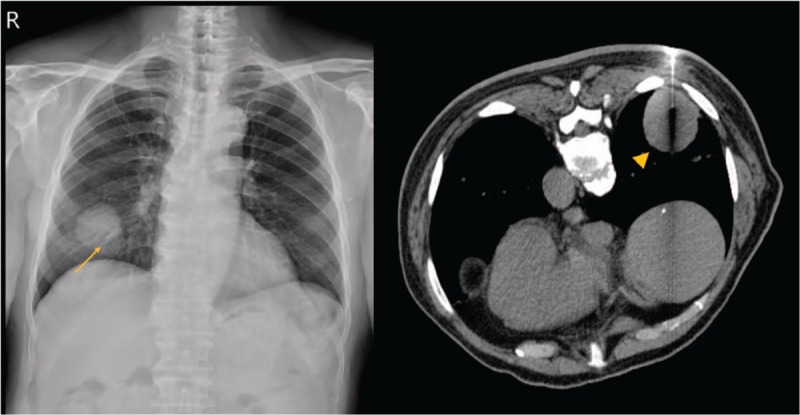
The chest X-ray showed opacity in right lower lung (arrow). Computed tomography (CT) scan revealed a mass of 5 × 4.1 cm in right lower lung (arrowhead).
